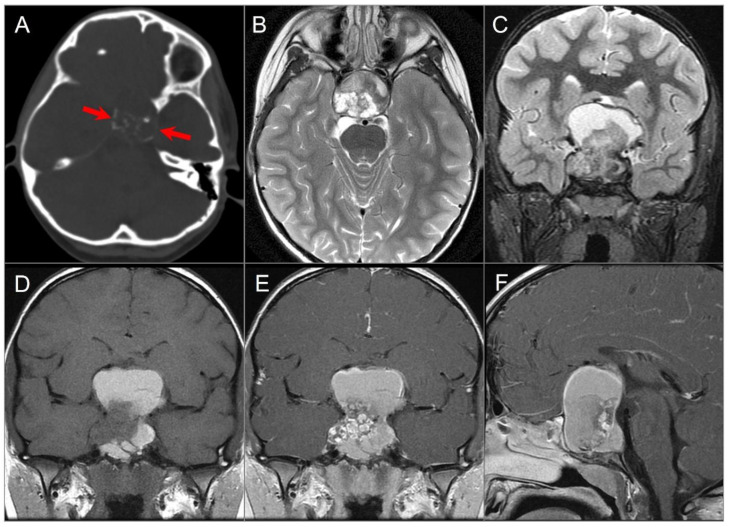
Adamantinomatous craniopharyngioma in a child. (A) CT image shows a partially calcified sellar and suprasellar mass (red arrows). (B) Axial and (C) coronal T2W, (D) coronal noncontrast T1W, and (E) coronal and (F) sagittal postcontrast T1W images show a complex mass with mixed cystic and solid components. Note: hyperintense T1 and T2 signal in the cystic components consistent with proteinaceous, hemorrhagic and/or cholesterol contents. The mass demonstrates intrasphenoidal and intraclival extension with mild prepontine bulge and suprasellar extension with mass effect on the optic chiasm and third ventricle. Heterogenous enhancement of the solid tumor components is noted.
